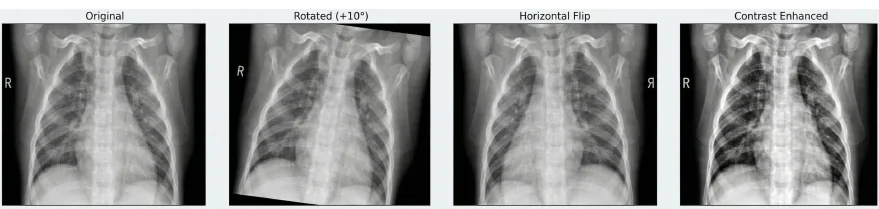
Augmented chest X-ray images with different transformations.
